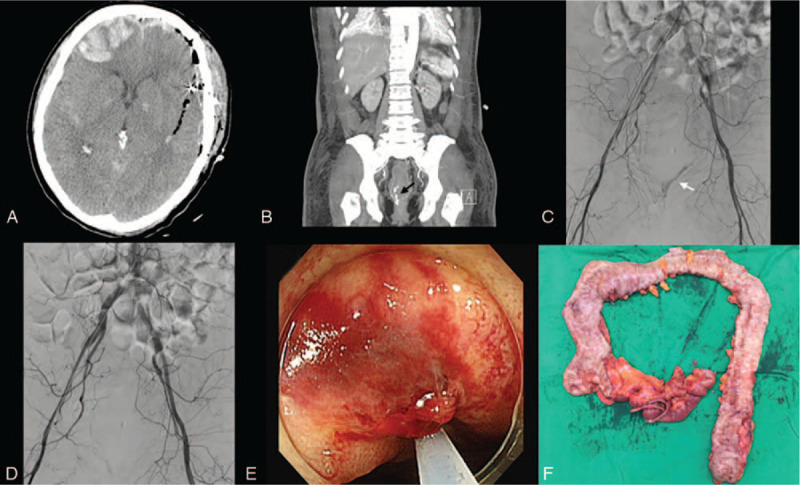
Case 1. A: Patient underwent craniotomy and removal of a hematoma due to traumatic intracerebral hemorrhage in the left temporal lobe. B: APCT revealed media extravasation at rectum (black arrow). C. Pre-angioembolization angiography shows extravasation of right middle rectal artery (white arrow). D: Extravasation of contrast media stopped following embolization with histoacryl and lipiodol. E: Following a recurrence of bleeding, colonoscopy reveled multiple ulcerative lesions with suspected exposed vessel. F: Total colectomy and end-ileostomy was performed for management of refractory hematochezia. APCT = abdomen/pelvis computed tomography.
